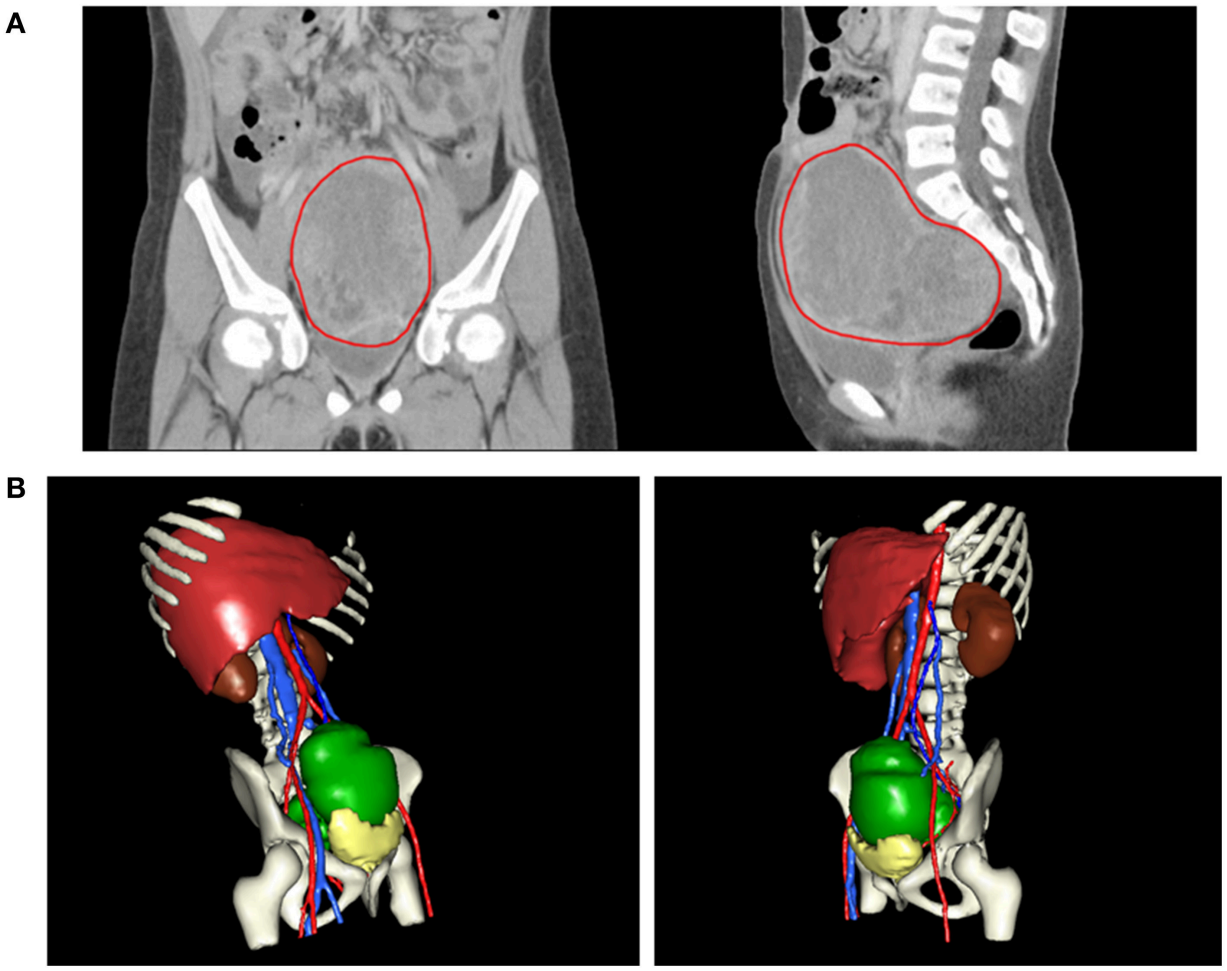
(A) CT revealing a large pelvic mass measuring 12 × 9.7 × 7.38 cm in size (circled by red line). (B) A three-dimensional virtual model created from 1.25-mm thin-slice CT images showing the tumor of 12 × 9.7 × 7.38 cm in size (green).
